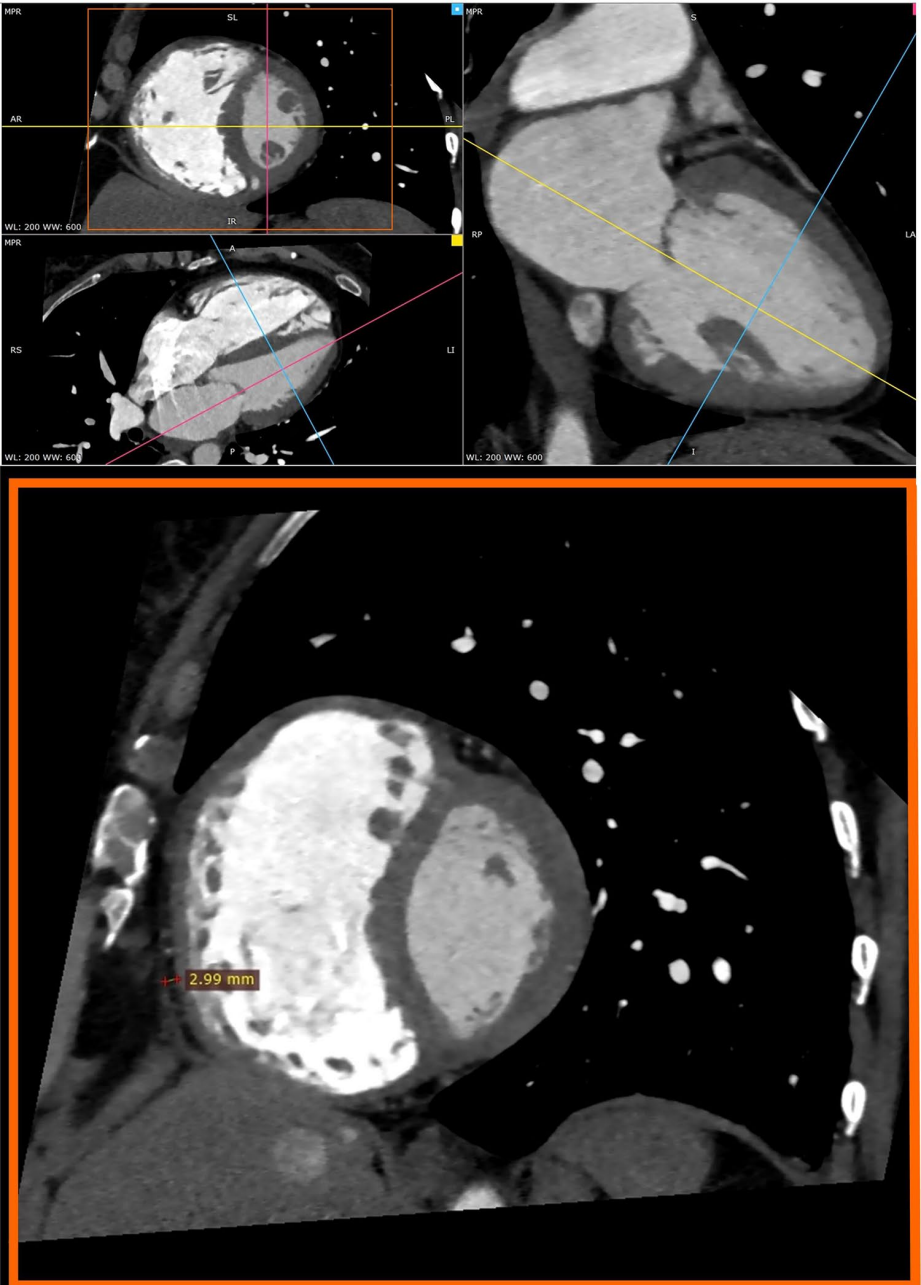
Assessment of the epicardial adipose tissue thickness in computed tomography angiography images
